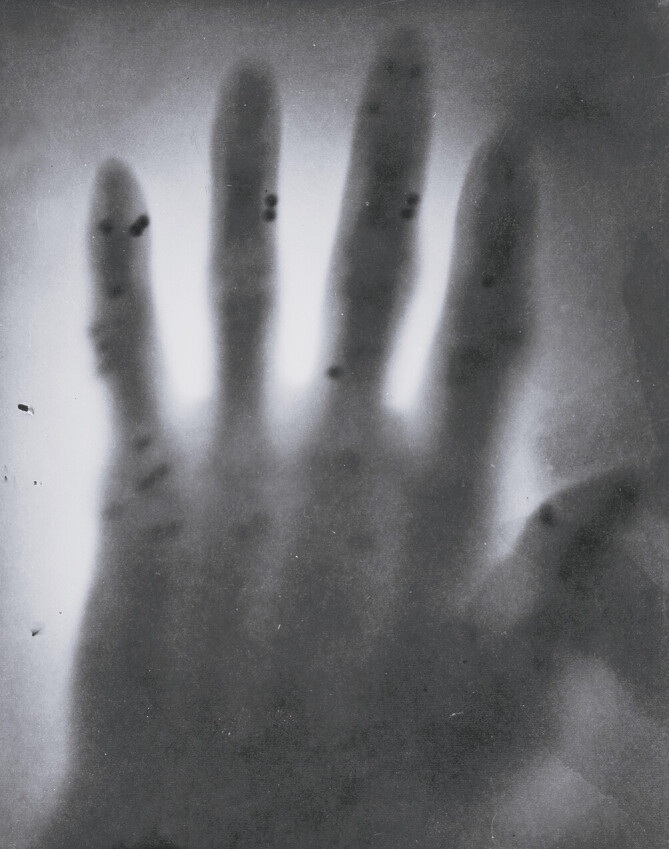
Print of the X-ray of Prescott Hall Butler’s left hand with gunshot acquired by Pupin at Columbia College, New York, on February 15,1896. Michael Idvorsky Pupin Papers. Rare Book & Manuscript Library, Columbia University in the city of New York. Available from:
